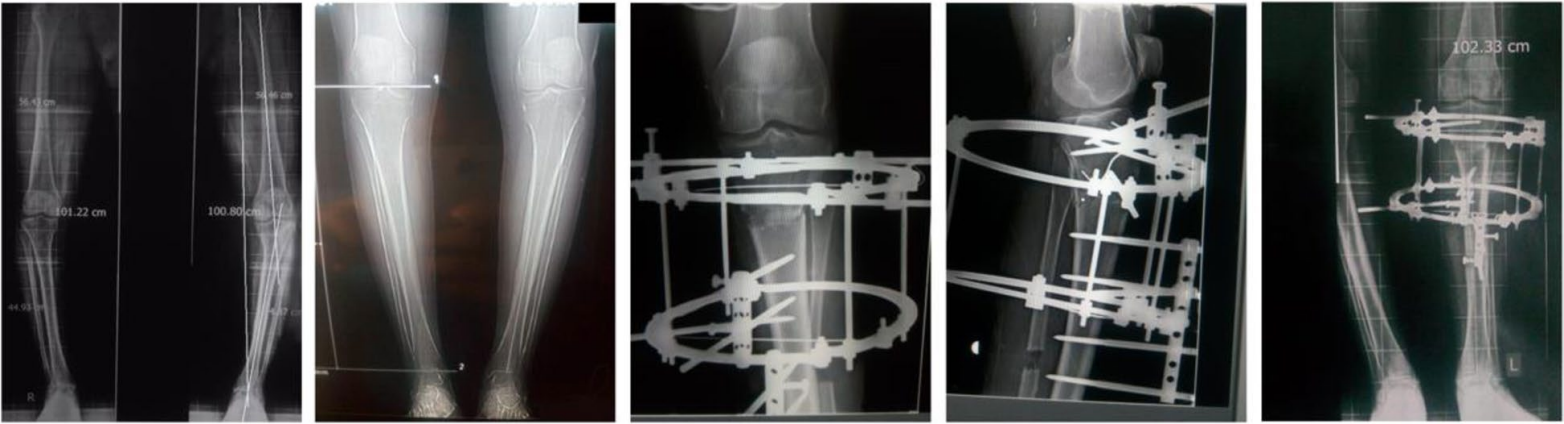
Pre- and post-operative radiographs showing acute correction of late onset tibia vara in 22-year-old male patient
